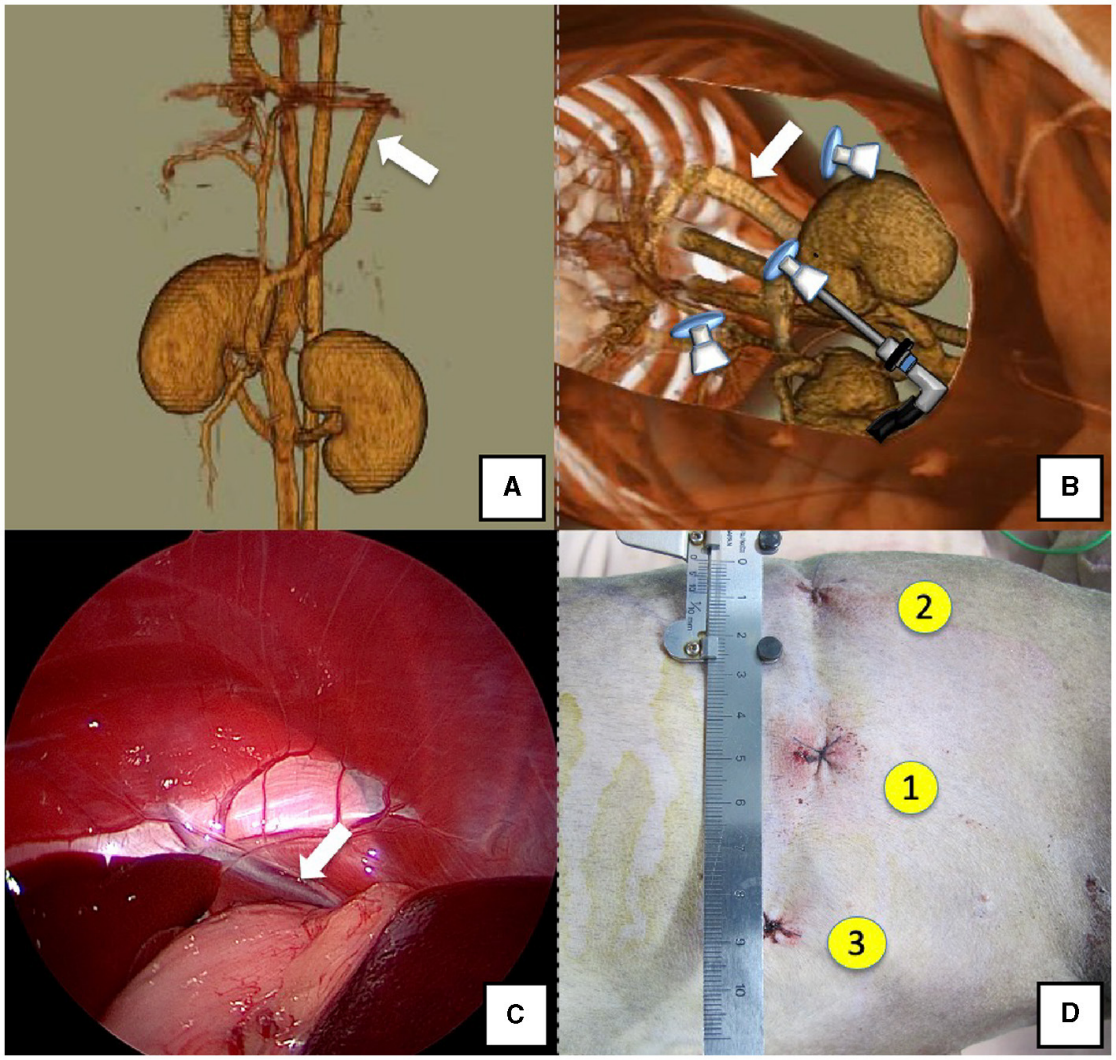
Spleno-phrenic shunt (white arrow). (A) 3D-CT angiography image. (B) 3D-CT image of the port locations from reconstructed right recumbent CT images. (C) Laparoscopic image of the shunt vessel and approach. (D) Actual surgical wounds and port placement order. There are surgical wounds for only three ports because the splenic vein was used percutaneously. 3D-CT, three-dimensional-computed tomography.
